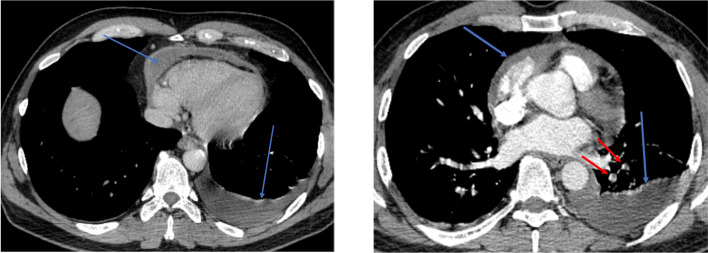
Chest CT scan: (A) notice the pericardial effusion, the left pleural effusion and (B) the bilateral, subsegmental pulmonary embolism (arrows).
